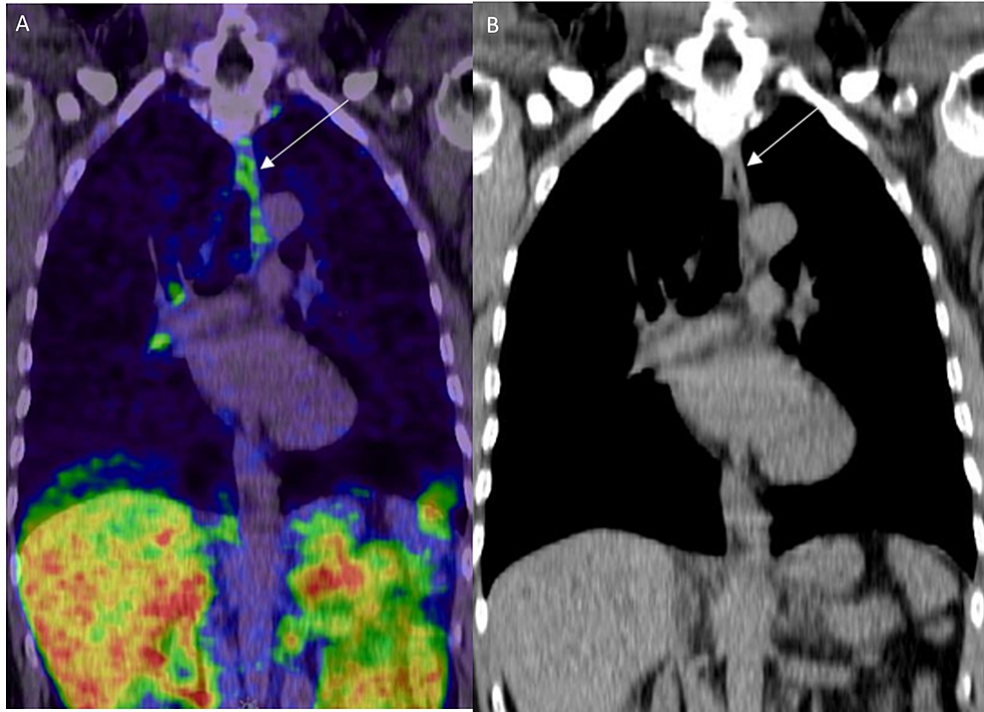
Benign esophageal PSMA uptake A: 68Ga PSMA PET/CT in coronal projection shows mild linear tracer uptake along the esophagus (white arrow) due to inflammation. B: Coronal CT image of the chest in the same patient shows the esophagus (white arrow) with no abnormal wall thickening.
